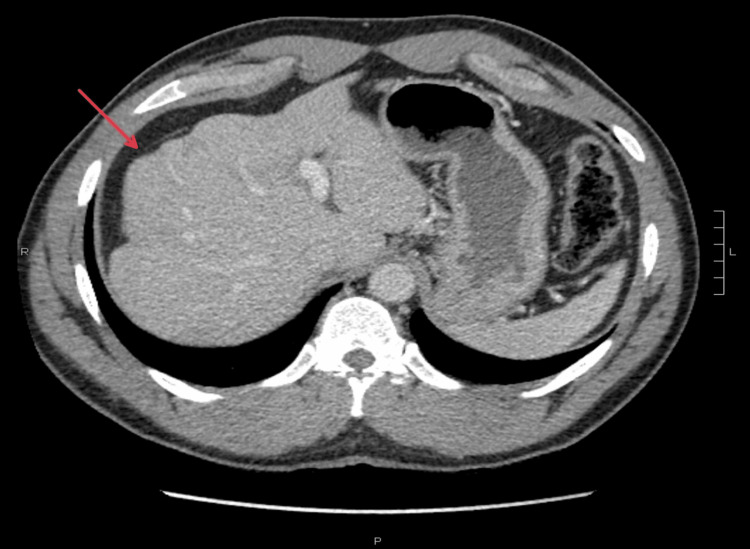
CT of the abdomen showing nodular liver contour with capsular retraction concerning for cirrhosis.
